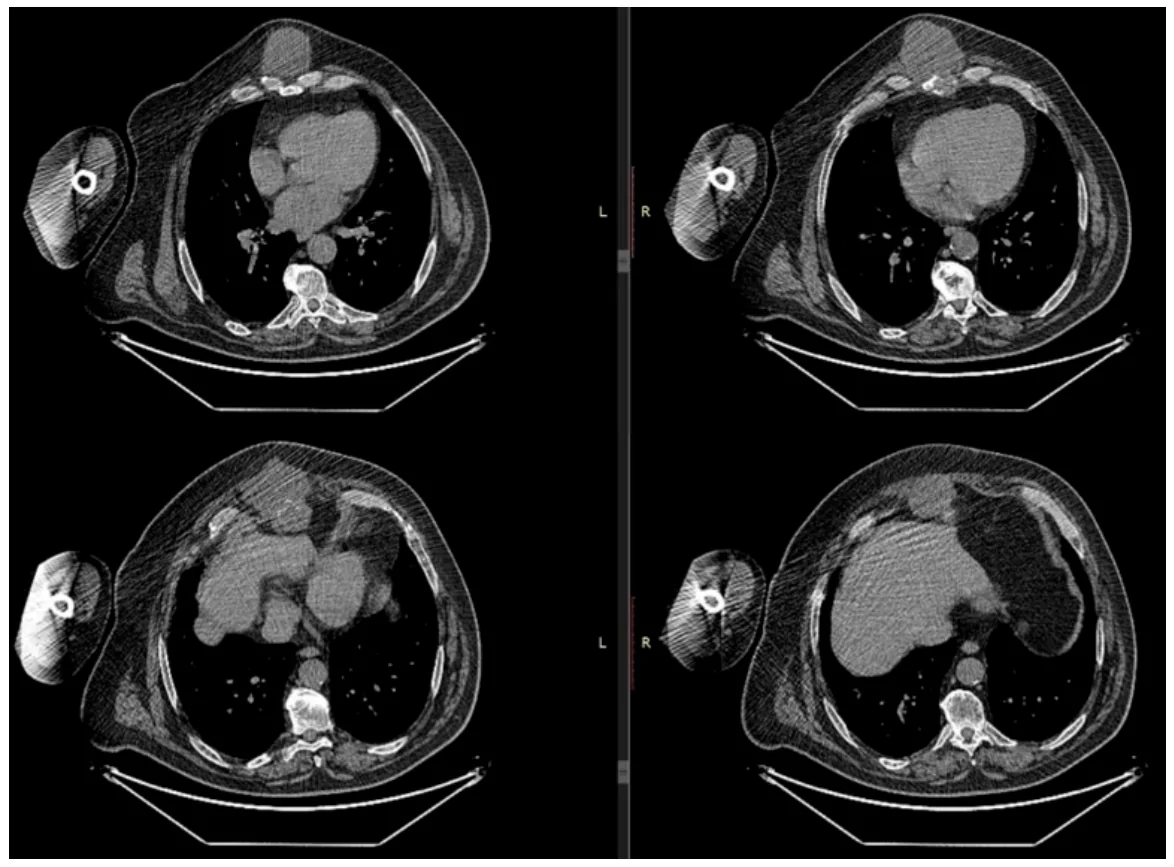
Preoperative axial CT images demonstrating the lower anterior chest wall lesion and its relationship to the thoracoabdominal junction.
